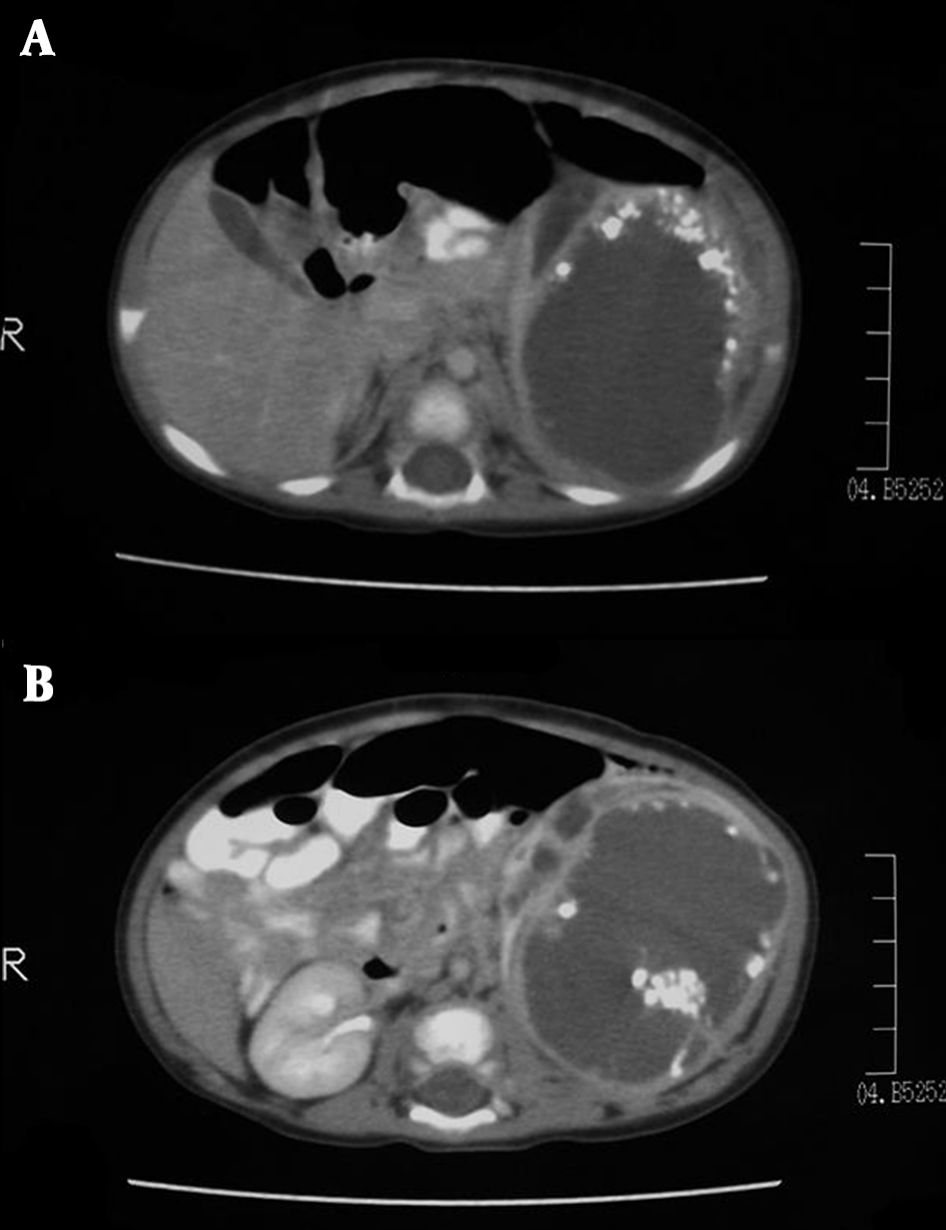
A, Peripheral coarse calcifications on CT. B, Calcifications are clustered in particular regions of the mass.
